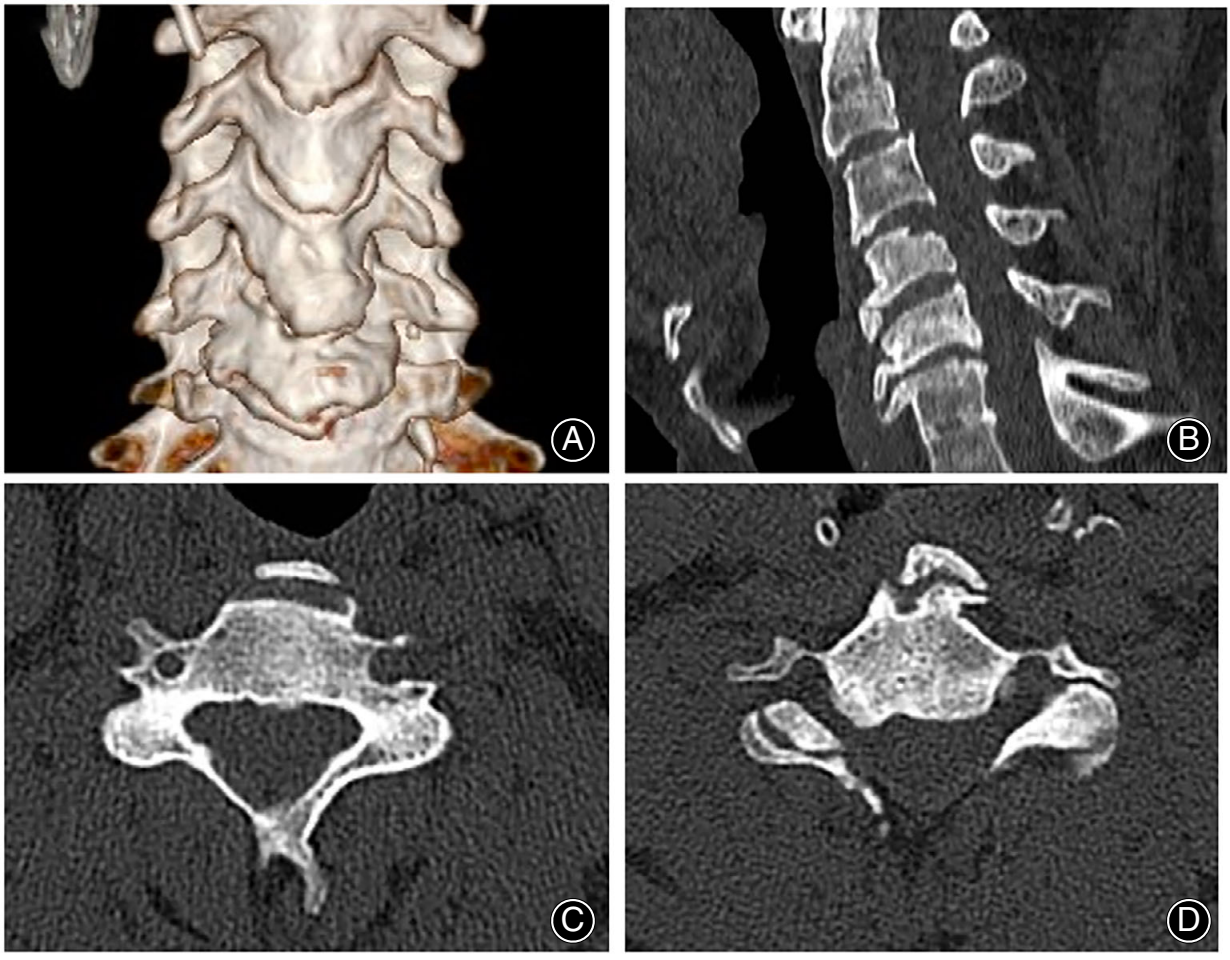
Follow‐up CT images. The bone channel almost disappeared 24 months after surgery, including the three‐dimensional view (A), sagittal reconstruction view (B), and axial plane view (C, D).
